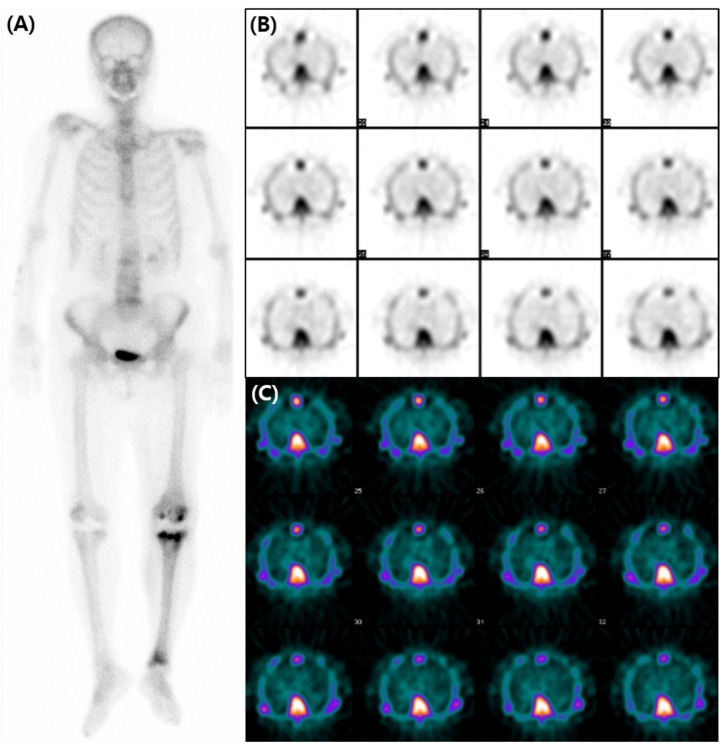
A subsequent bone scan using ^99m^Tc-3,3-diphosphono-1,2-propanodicarboxylic acid (DPD) was obtained three weeks later including SPECT images for assessment of transthyretin-type cardiac amyloidosis (CA). The decision was influenced by the accumulating evidence that favored the use of ^99m^Tc-DPD over ^99m^Tc-HDP for detecting CA [2,3,4], and by the necessity of SPECT images. However, no perceptible radiotracer accumulation was observed in the subsequent ^99m^Tc-DPD scan (A). Additionally, no perceptible radiotracer uptake was noted in the myocardium on the axial SPECT images (B,C). An echocardiogram revealed preserved ejection fraction of 60% without any abnormal wall thickness or abnormal wall motion. No abnormality was found in serum free light chain, serum and urine protein electrophoresis/immunofixation data. Upon thorough review of her medical history, her hemoglobin level was 8.7 g/dL, and she was diagnosed with iron-deficient anemia. We also observed that a total of 1000mg of ferric carboxymaltose had been intravenously administered the day before the ^99m^Tc-HDP bone scan. Hence, it is assumed that the diffuse and temporary cardiac activity was a result of transient iron overload. Bone scan using ^99m^Tc-DPD or ^99m^Tc-PYP is known for its several advantages in the diagnosis in CA. They not only have high sensitivity and specificity but also provide a non-invasive, whole-body evaluation and are readily accessible [5,6]. Currently, multidisciplinary experts in cardiovascular imaging and cardiac amyloidosis recommend including bone scans for diagnosis of CA [7]. However, caution should be exercised in the interpretation of myocardial uptake in a bone scan, as altered biodistribution of radiotracer can occur in various medical conditions including iron overload [8,9,10,11,12]. As bone scans are widely used in the diagnosis of CA, we present and share this image in order to avoid possible future misinterpretation.
